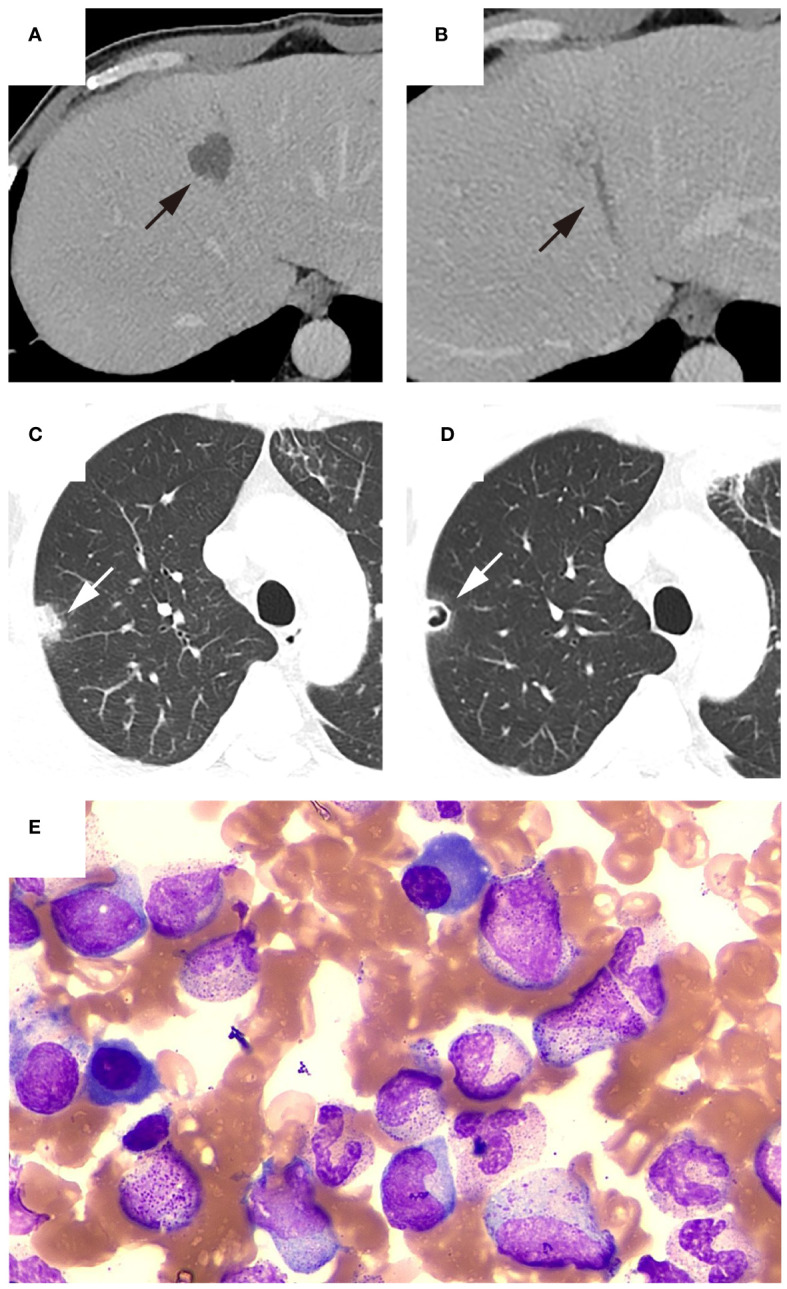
Representative patient with thrombocytopenia. The patient was admitted to the hospital with a high fever and chills lasting for two days. CECT showed ((A), black arrow) liver abscess with ((B), black arrow) thrombophlebitis of middle hepatic vein, and ((C), white arrow) chest CT revealed a nodule in the right upper lobe of the lung. A follow-up chest CT five days after admission showed ((D), white arrow) the presence of a new cavity within previously identified lesion in the right upper lobe. Laboratory tests indicated a platelet count of 36×10^9/L. Bone marrow aspiration was performed to determine the cause of thrombocytopenia. (E) Bone marrow smear showed megakaryocyte hyperplasia and no thromocytogenic megakaryocyte, indicating poor platelet production. Subsequently, blood culture results were positive for Klebsiella pneumoniae. Blood glucose monitoring after admission revealed poor glycemic control. During hospitalization, the patient received antibiotics and hypoglycemic treatment and the platelet count recovered to 103×10^9/L three days after admission. The patient was discharged after 21 days of hospitalization due to reduced abscess size and significant decrease in inflammatory markers.
